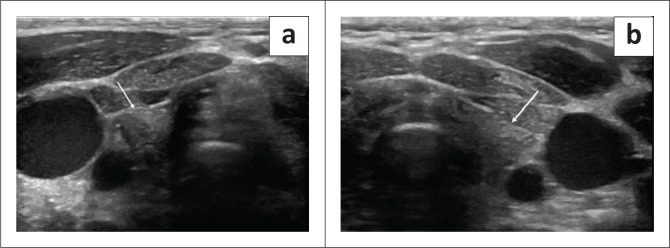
Axial ultrasound images of the thyroid gland showing a hypoplastic right lobe (arrow in a) and left lobe (arrow in b). The isthmus is not visible in the midline.
